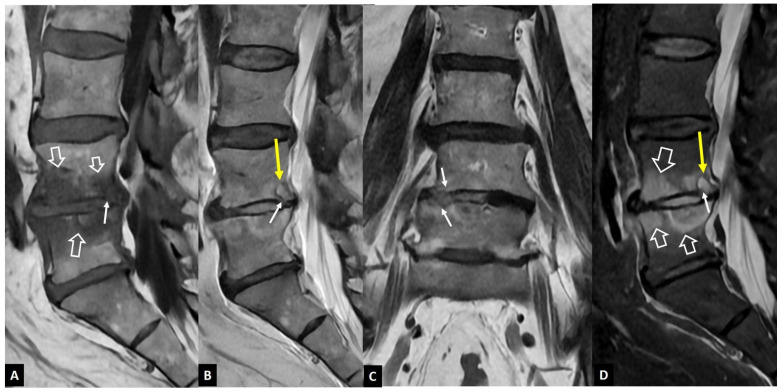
A 73-year-old male patient with *Staphylococcus aureus* infectious spondylodiscitis. Sagittal T1-w (A) and T2-w (B), coronal T2-w (C), and sagittal STIR (D) MR images showing the bone marrow edema at L4 and L5 (open arrows), the endplate cortical disruption (small arrows), and the abnormally high signal within the disc on T2-w and STIR images. A small intraosseous abscess formation is also seen (long arrows).
